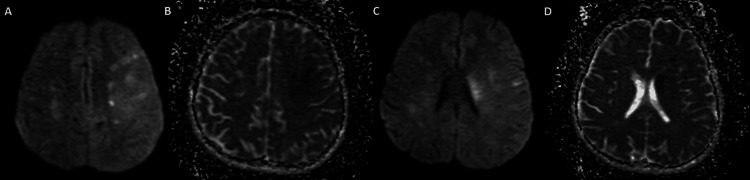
Multiple left cerebral internal border zone infarcts demonstrating restricted diffusion These appear hyperintense on DWI (A, C) with corresponding ADC images (B, D) showing dark signals. DWI: diffusion-weighted imaging; ADC: apparent diffusion coefficient
